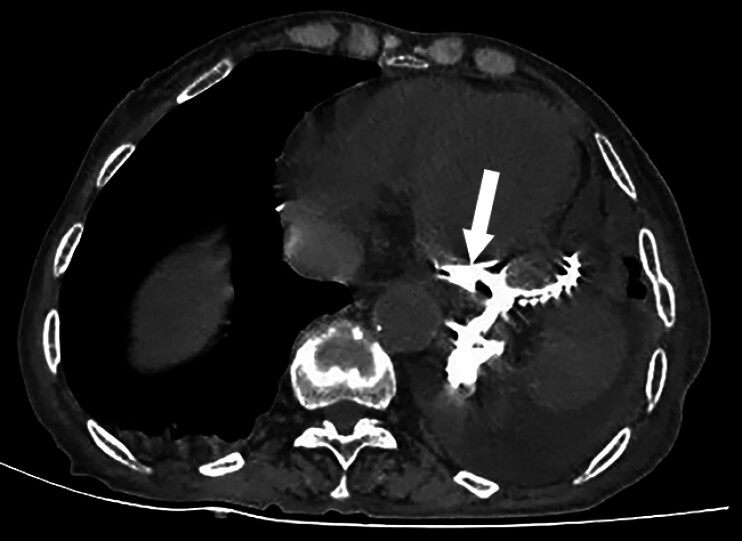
A chest computed tomography scan revealed a linear contrast shadow posterior to the left heart.
